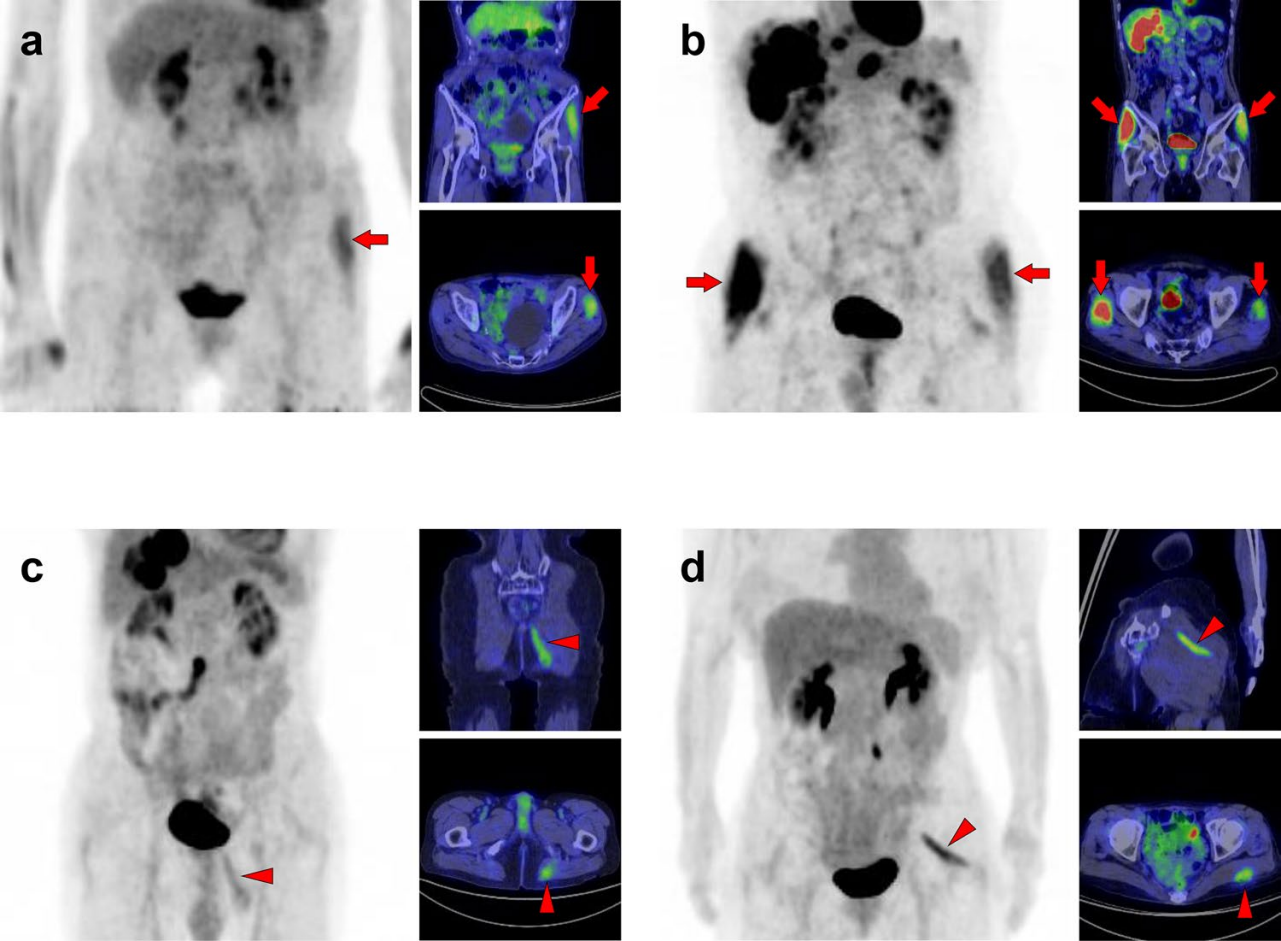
Gluteus medius muscle and gluteus maximus muscle.* The gluteus medius (a, b, arrows), where non-pathologically increased [^18^F]FDG uptake is frequently observed among the gluteus, is composed of anterior, middle, and posterior parts. The anterior parts located in the lateral side are most often found to be with uptake. In contrast, the gluteus maximus muscle is often visualized entirely with other skeletal muscles after exercise or in a high-insulin state. However, localized uptake may be observed limited in the lower parts located medially (c, arrowheads). Since the gluteus maximus muscle is often chosen for intramuscular injection, we should consider the possibility of a medically induced uptake (d, arrowheads)
